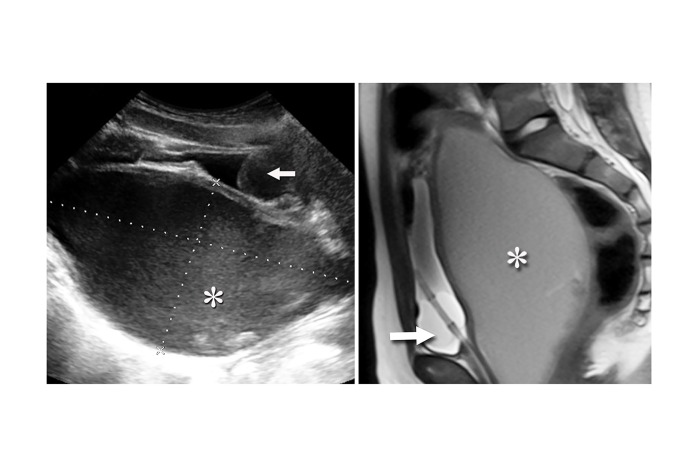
Long axis transabdominal sonographic view (left) of the patient’s abdomen revealing intrauterine low-level echogenic material (asterisk) communicating with the vaginal vault and a Foley catheter within a decompressed bladder (arrow). Sagittal magnetic resonance image (right) demonstrating fluid-filled distention (asterisk) of the patient’s uterus and vagina to the level of the introitus and a Foley catheter within the decompressed bladder (arrow).
